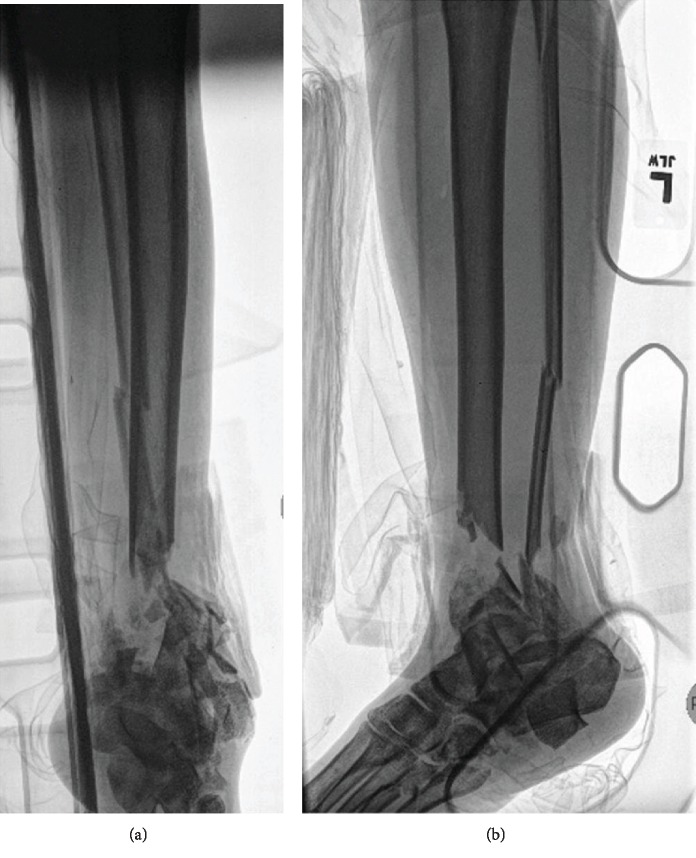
Anteroposterior (a) and lateral (b) radiographs taken at the time of the original injury demonstrating distal tibia bone loss and multiple foot fractures.
